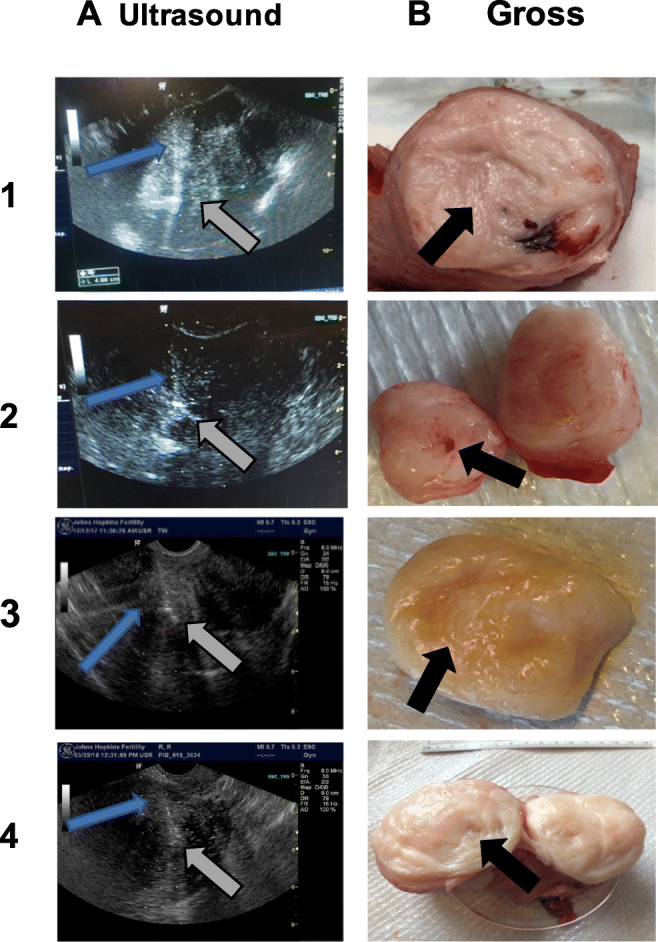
Representative images of the ultrasound guided study drug injection (Column A), gross hemi-section of the fibroid tissue (Column B) injected with various doses of collagenase group1, 1.16 mg (row 1), and group 2 dose 1, (row 2), dose 2, (row 3), and dose 3, (row 4) with 1.68, 3.35, and 5.028 mg as the maximum doses, respectively. The blue arrows mark the needle, grey arrows mark the study drug, and the black arrows mark the area of digestion by the study drug in the hemisected fibroid sample. The areas of digestion were visibly darkened and softened (black arrows, rows 1, 3, 4), and sometimes completely liquefied, as in the hole marked row 2 (black arrow)
